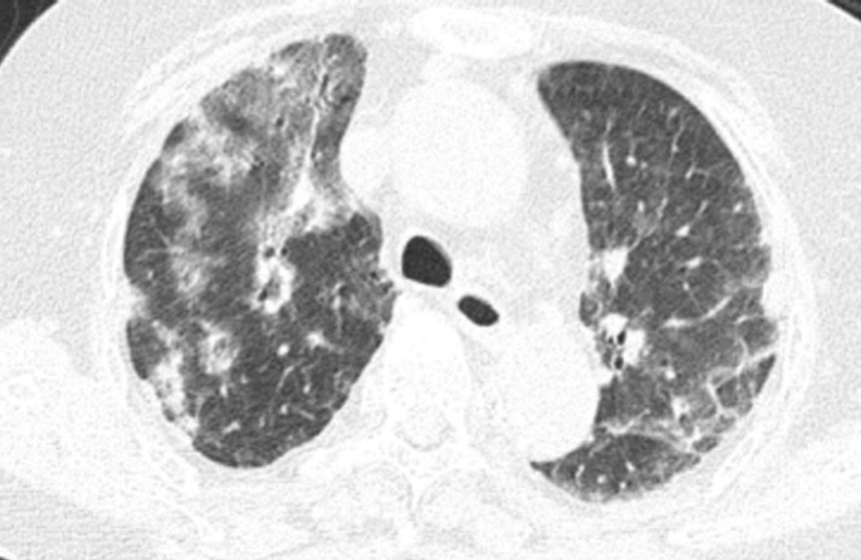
The chest images of this patient. The CT scan showed diffused effusion and GGO in both lungs.
